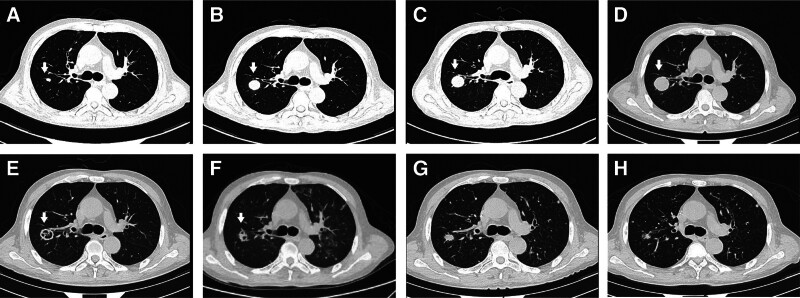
The lung CT scans of the upper lobe of the right lung at different times. (A) December 15, 2023. (B) January 2, 2024. (C) January 11, 2024. (D) January 25, 2024. (E) March 4, 2024. (F) April 3, 2024. (G) June 18, 2024. (H) October 10, 2024. CT = computerized tomography.
